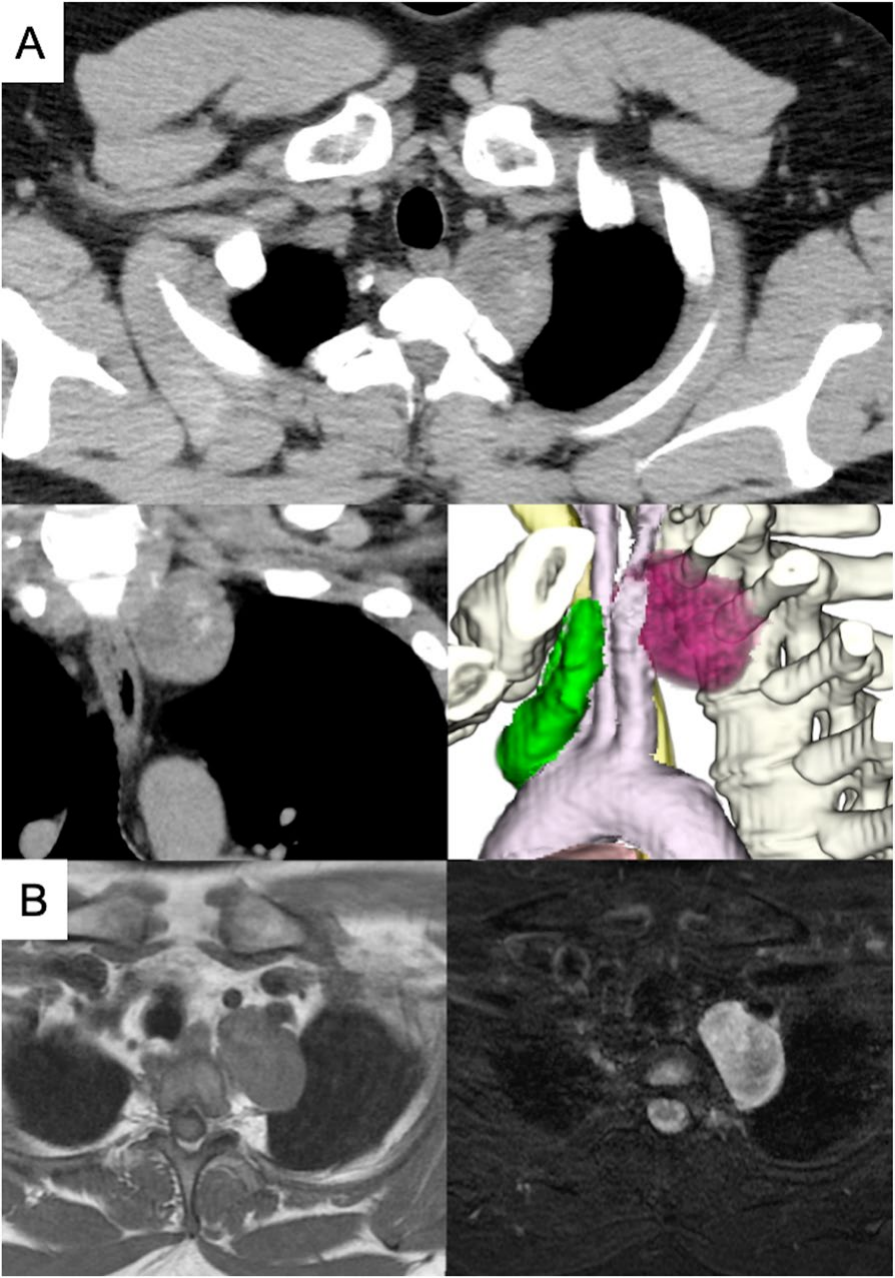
A Chest computed tomography (CT) shows a 5.0-cm mass in the thoracic apex. The tumor is adjacent to the left subclavian vessels, common carotid artery, and first vertebral body. B Magnetic resonance images show T1 iso intensity and T2 high intensity
